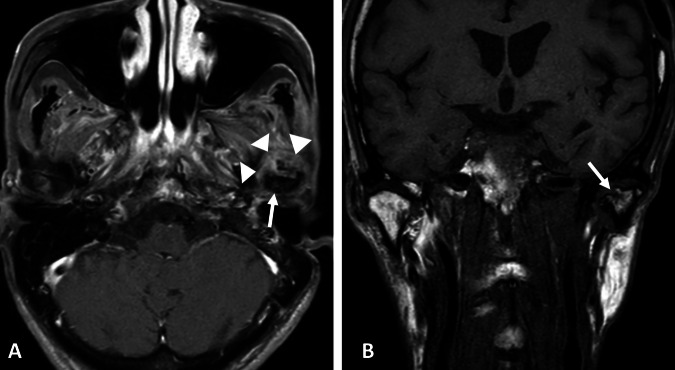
Post-treatment axial fat-suppressed contrast-enhanced T1W (A) and coronal contrast-free T1W (B) MRI of a patient with nasopharyngeal carcinoma (NPC) treated with RT over 20 years ago. The enhanced soft-tissue-like features (arrow heads) around the left mandibular condyle (arrows), the poorly marginal areas of the left mandibular condyle (arrows) and the decreased size of the left mandibular condyle (arrows) compared to the contralateral side could be sequelae of post-RT-induced inflammatory changes
